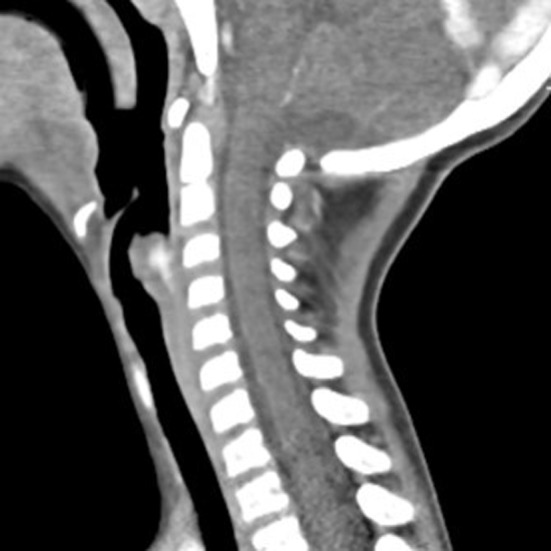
Computed tomography angiography showing an expansive lesion with soft tissue density, causing a reduction of about 60% of the laryngeal lumen, with a contrast enhancement effect in its central portion
